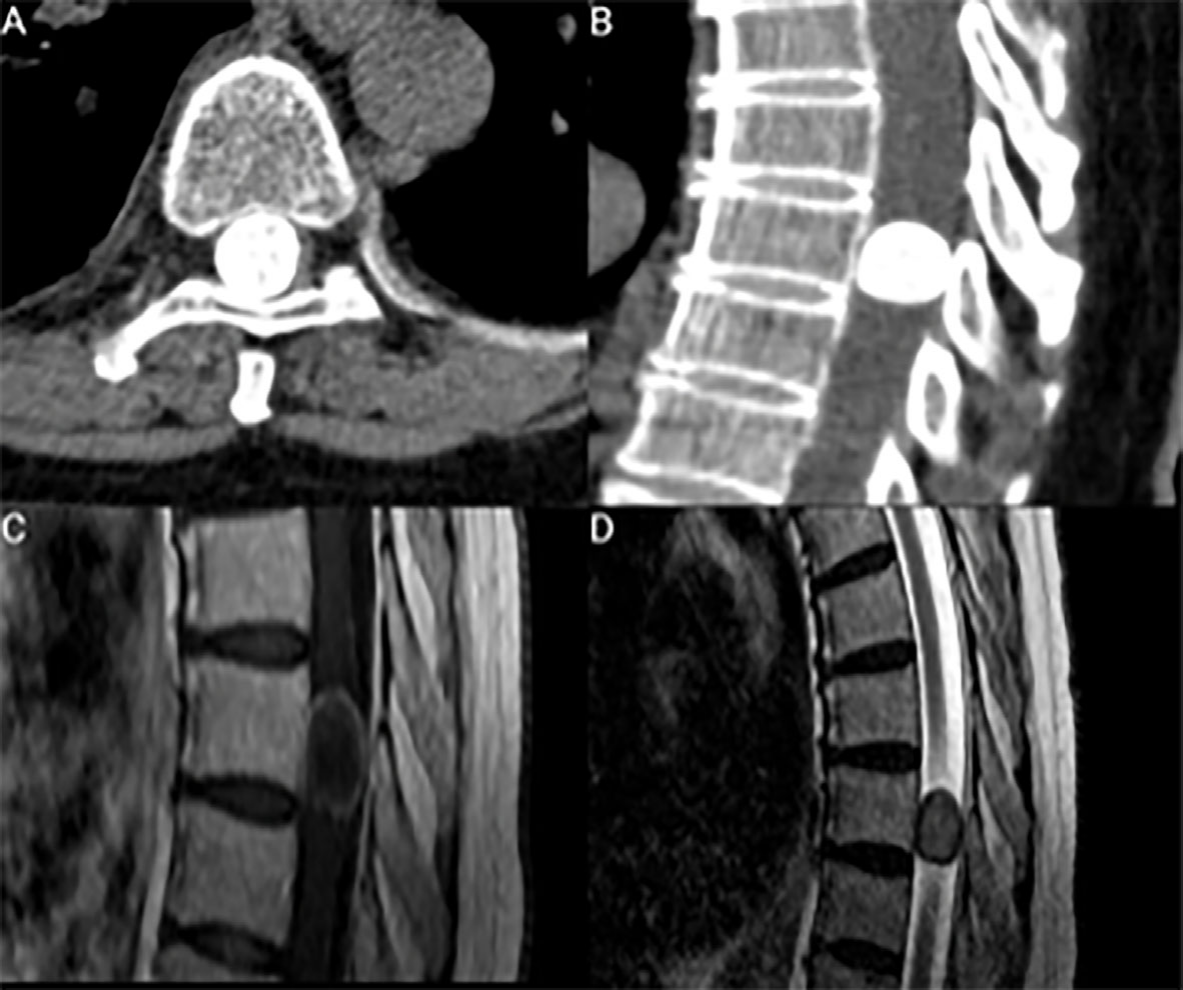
(A, B) Axial and sagittal CT scans showing a representative case with a gross calcification of a thoracic spinal meningioma. (C, D) represent sagittal T1-weighted Gd-enhanced and T2-weighted MR-images. (C) shows a heterogeneous ring-enhancing lesion because of the gross calcification.
